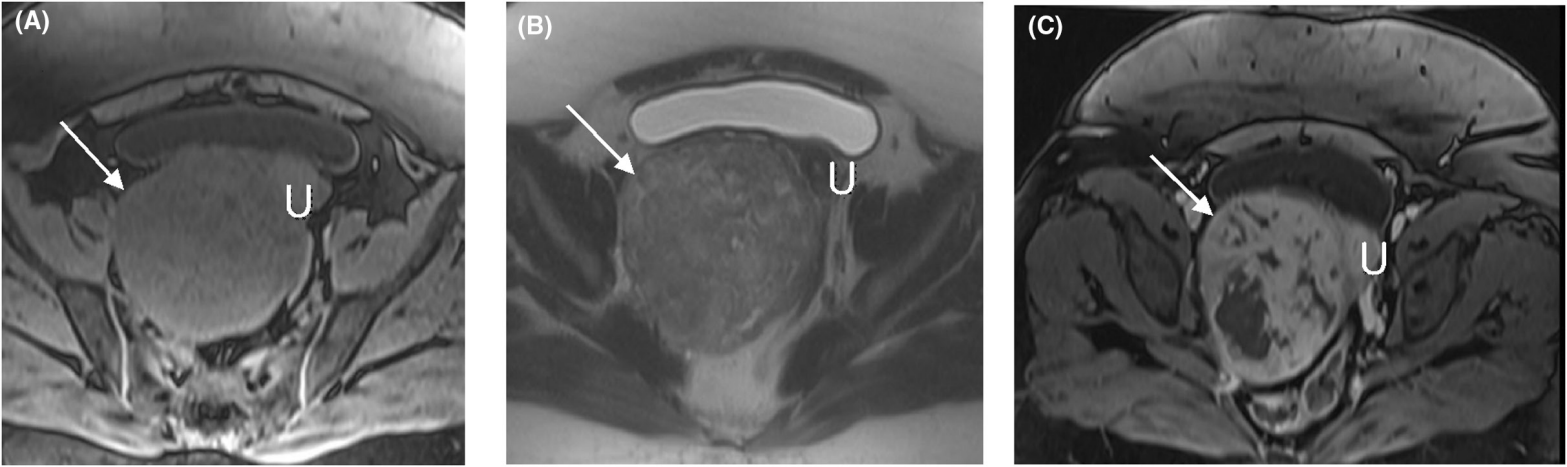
Axial T1‐weighted fat‐suppressed (A), T2‐weighted (B), and gadolinium‐enhanced T1‐weighted fat‐suppressed (C) MR images show a large relatively well‐defined, homogeneous, non‐fat‐containing solid intra‐abdominal mass that is isointense to skeletal muscle on T1‐weighted images (arrows on A), heterogeneously T2 hyperintense (arrows on B), and heterogeneously enhanced (arrows on C). Uterus = U on A–C.
